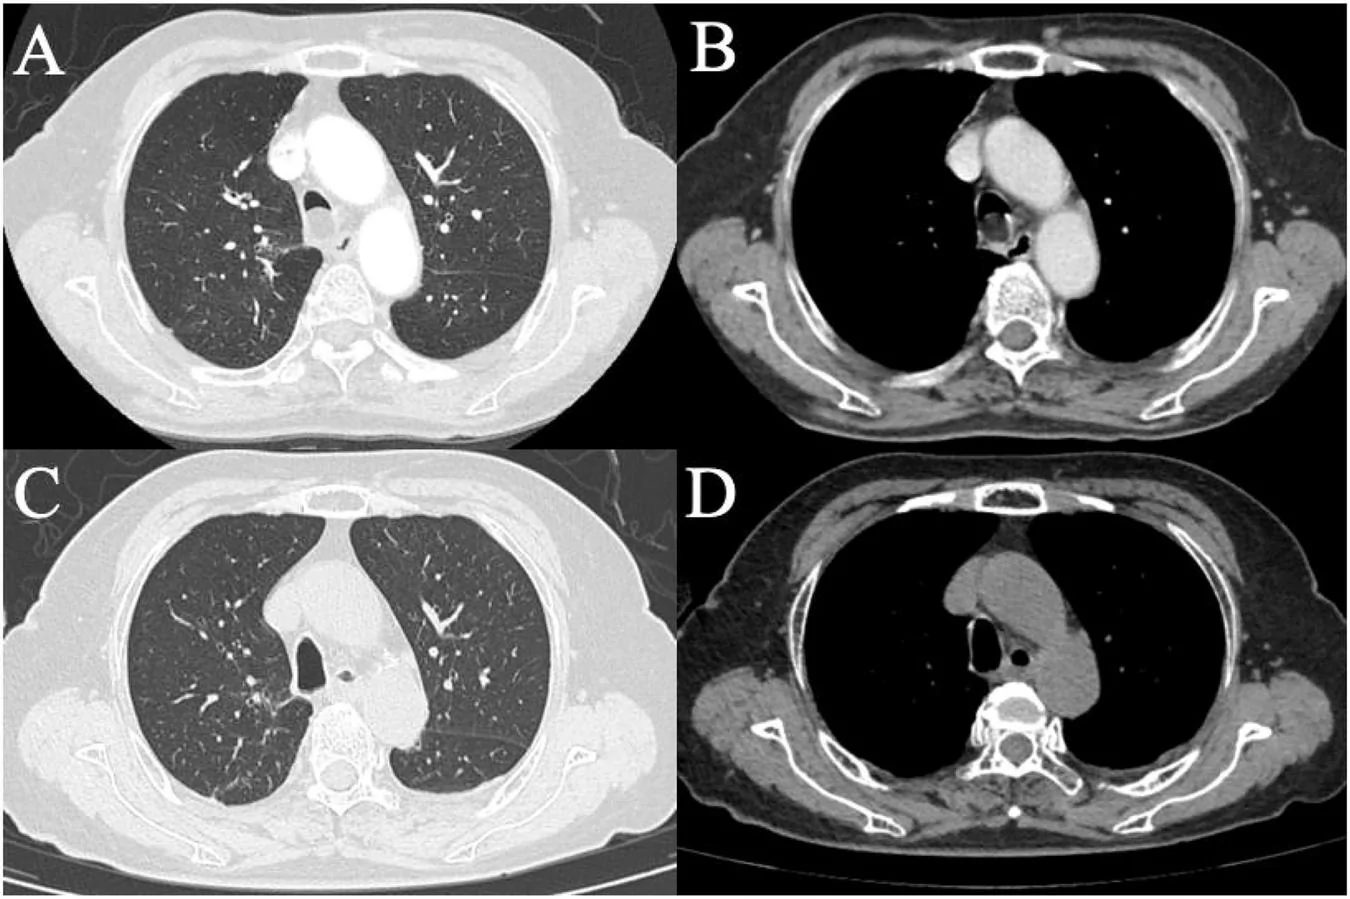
Chest CT images before and after treatment. (A) Preoperative contrast-enhanced chest CT (lung window setting) demonstrating a distal tracheal mass causing marked luminal narrowing. (B) Preoperative contrast-enhanced chest CT (mediastinal window, venous phase) showing a well-defined low-attenuation intraluminal lesion without appreciable enhancement. (C) Postoperative non-contrast chest CT (lung window setting) demonstrating restoration of tracheal patency following tumor removal. (D) Postoperative non-contrast chest CT (mediastinal window setting) confirming complete removal of the lesion without residual intraluminal abnormality.
